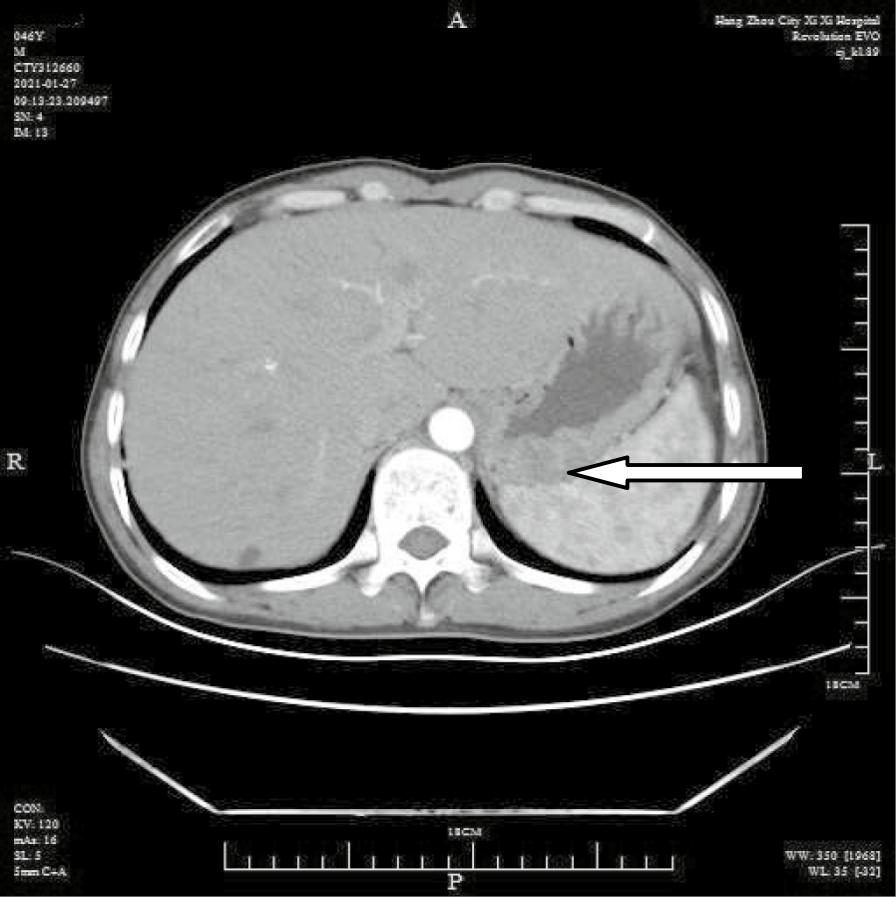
Thick stomach in CT before the treatment
